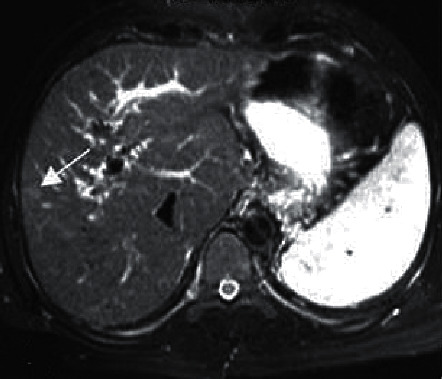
An MRI image of a male HCC patient (aged 50 years old).
